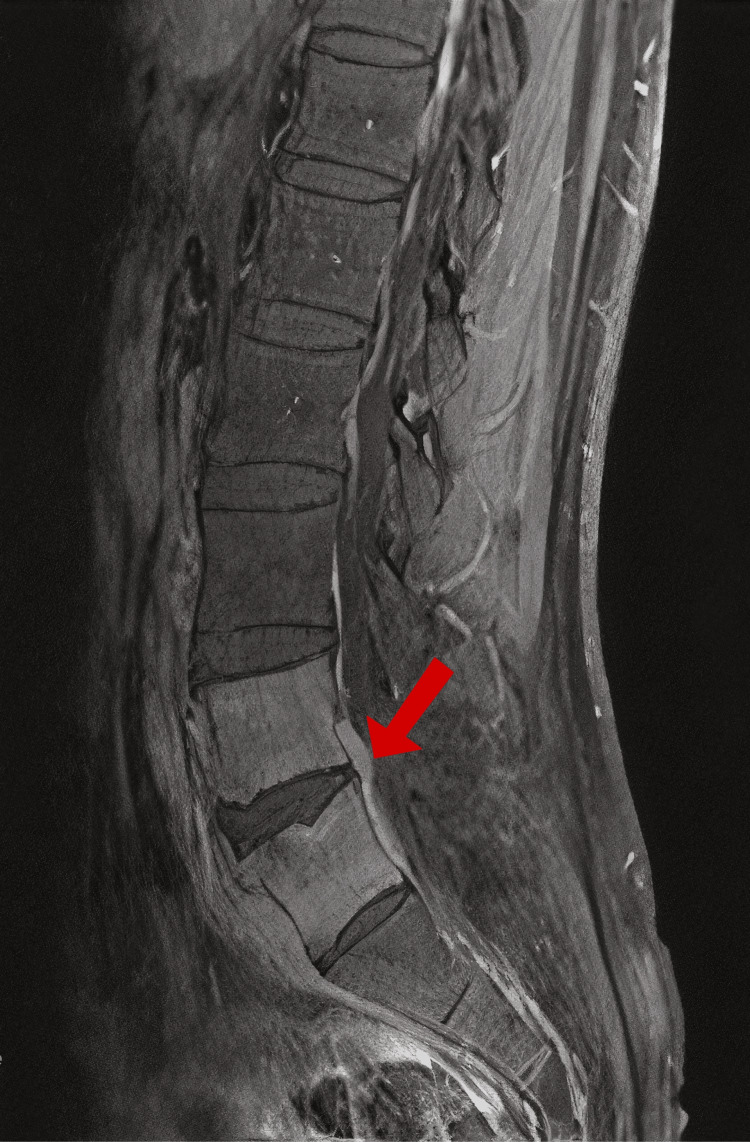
MRI lumbar spine, December 2, 2024 MRI: magnetic resonance imaging Sagittal T2-weighted MRI of the lumbar spine demonstrating persistent hyperintense signal in the L4-L5 intervertebral disc and adjacent vertebral endplates with surrounding epidural/paravertebral soft-tissue thickening (arrow)
